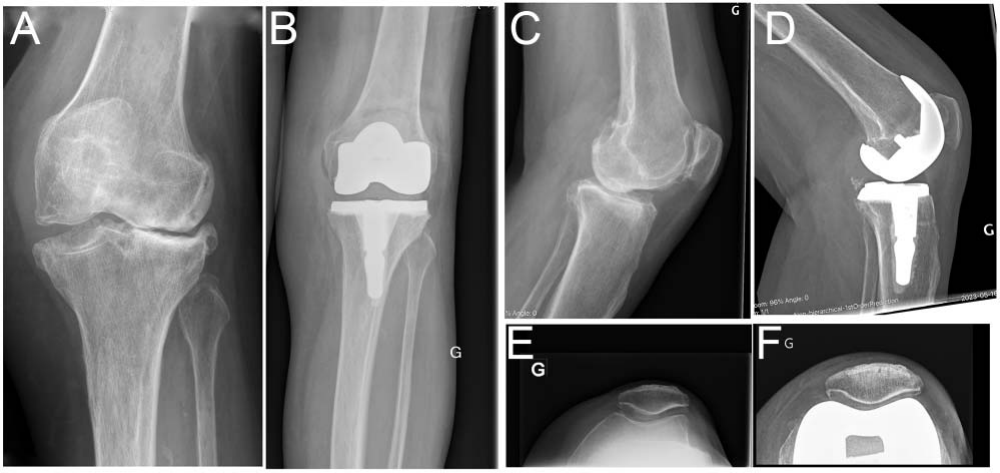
Valgus with global instability. (A and C) Preoperative radiographs of an 80-year-old female with a left knee in severe valgus (mechanical HKA of 9° valgus). The knee was grossly loose both medially and laterally upon clinical examination but had a firm end feel. (B and D) Using the rKA protocol, we resurfaced the medial femoral condyle (implant thickness from zones of intact cartilage) and removed 5 mm from the subchondral bone on the lateral compartment to reduce the femoral valgus from 7 to 5°. To compensate for the soft tissue hyperlaxity and minimize the polyethylene thickness, we under-resected by 3 mm the tibial side (7 mm from zones of intact cartilage for a 10 mm implant). Tibial cut angulation was 3° for an arithmetic HKA of 2° valgus. MCL laxity was estimated to be 4–5 mm at 10° of flexion, and a semi-constrained insert was used in combination with a short, cemented stem.
